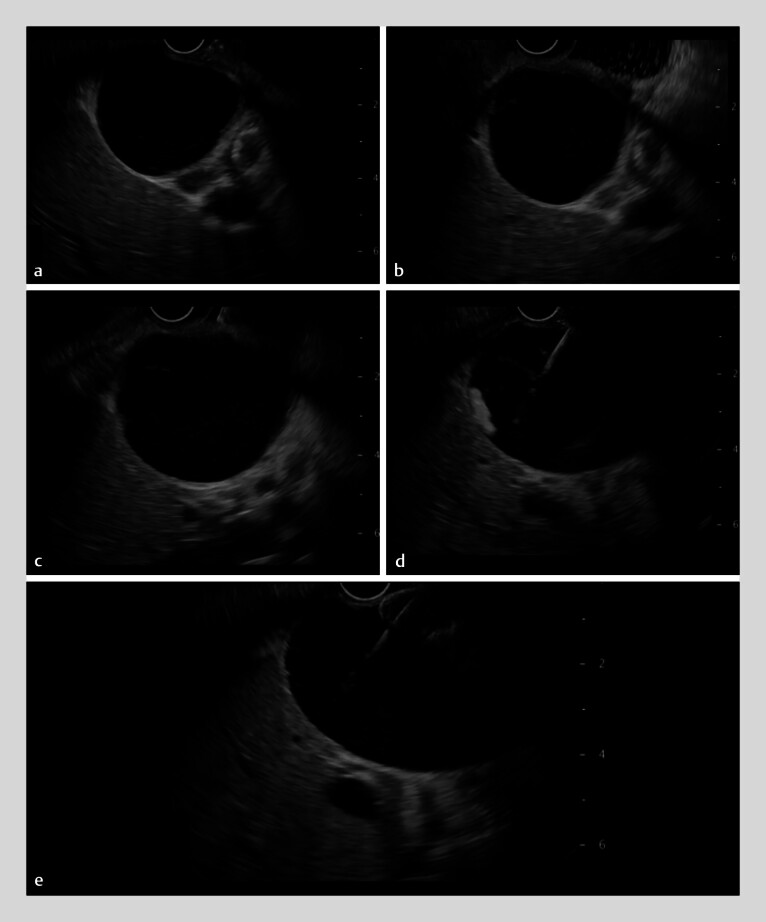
Endoscopic ultrasound images showing:
